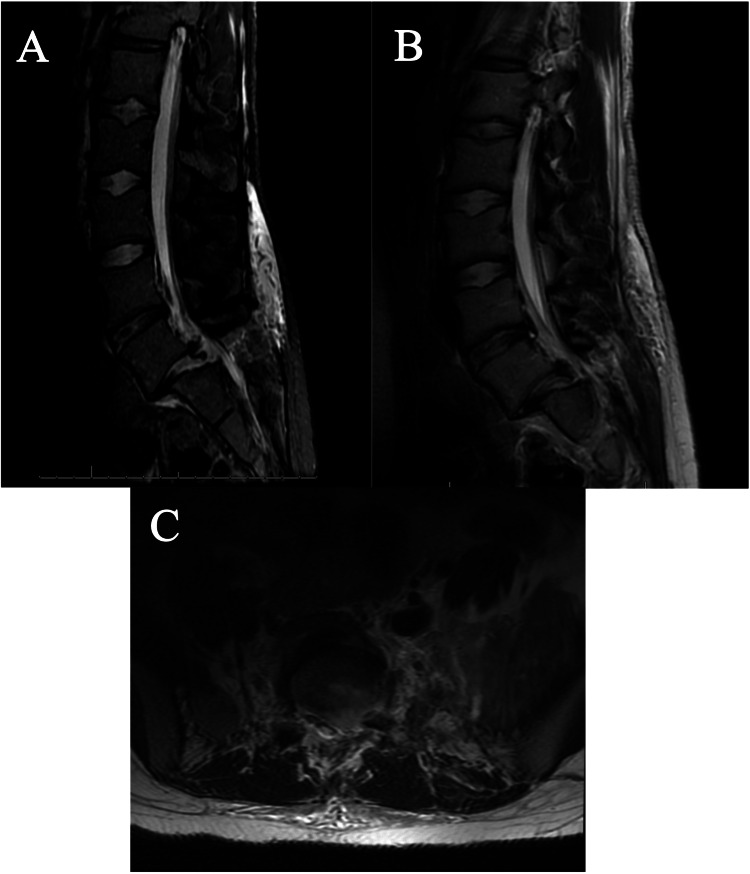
Pre-operative MRI of the lumbar spine (A) Sagittal view of lumbar MRI STIR sequence revealing L5 on S1 traumatic anterior spondylolisthesis with disruption of the PLL and extensive edema within the posterior ligamentous complex with associated widening of the L5/S1 interspinous space representing ligamentous disruption, (B) sagittal view of lumbar MRI T2 sequence again revealing L5 on S1 traumatic anterior spondylolisthesis with disruption of the PLL and posterior ligamentous complex in additional L5/S1 spinal canal stenosis, and (C) axial view of lumbar MRI T2 sequence showing the vertebral body and superior articular processes of S1 without the L5 inferior articular processes due to traumatic dislocation causing severe spinal stenosis.
